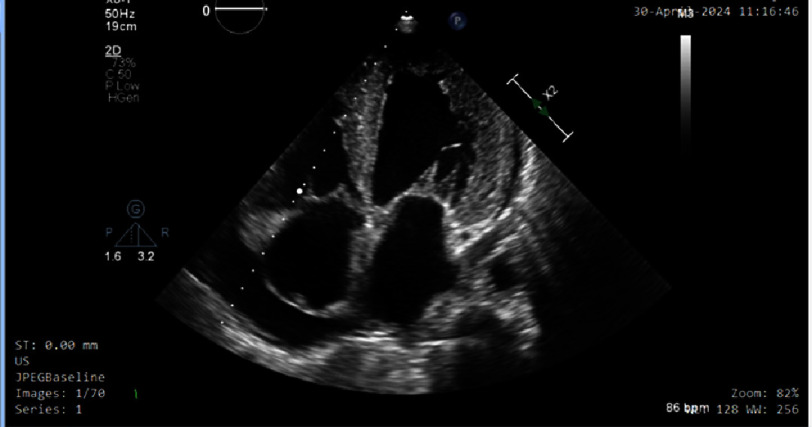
Two-dimensional transthoracic echocardiographic demonstrating concentric left ventricular hypertrophy.
